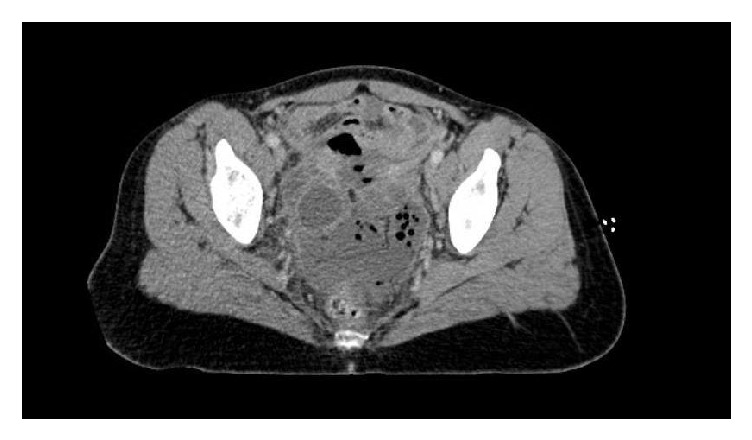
Axial CT image demonstrating abscess at vaginal cuff.
